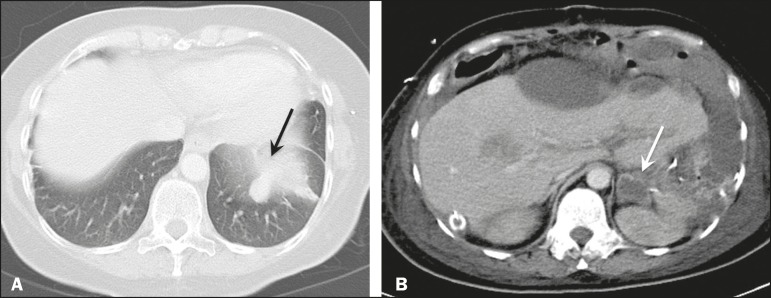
Follow-up CT scans of two different patients showing metastases to the lung (arrow in A) and to the left adrenal gland (arrow in B).
